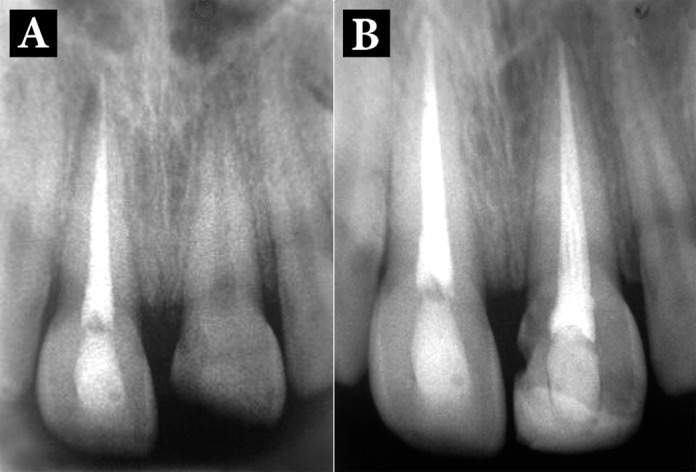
Follow-up radiographic images; A) One month; B) One year
